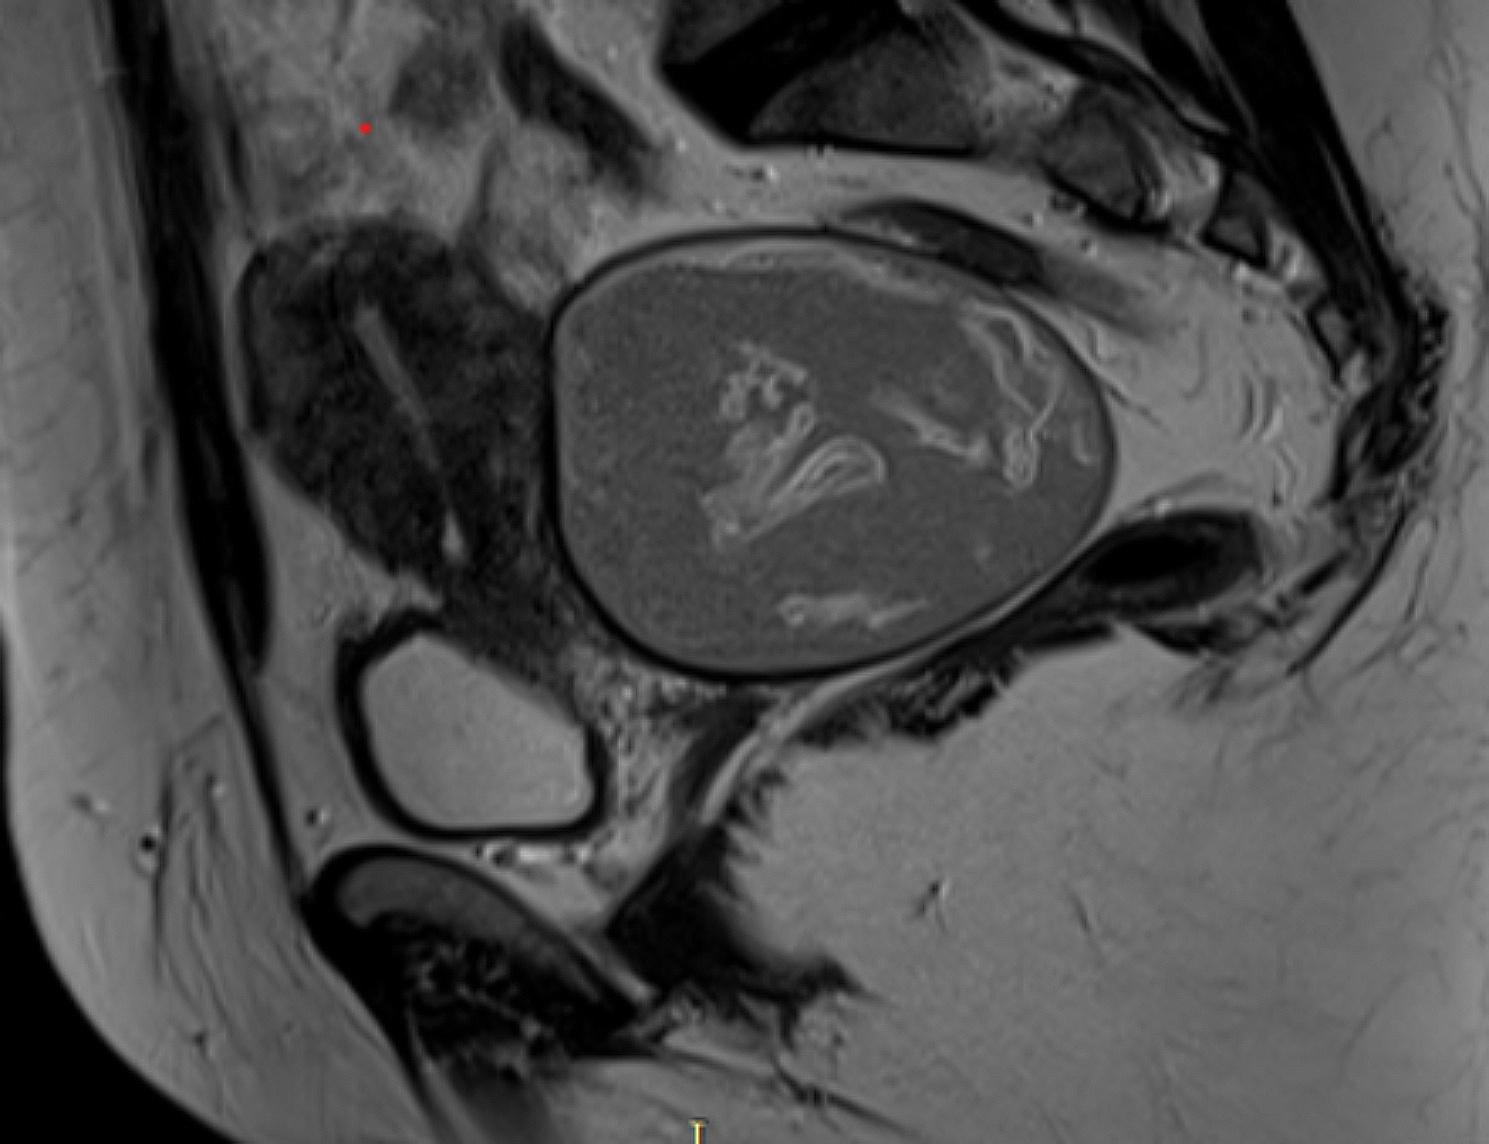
A pelvic MRI has demonstrated a voluminous pelvic cyst retro uterine, seat of serpiginous structures which not enhanced by gadolinium
